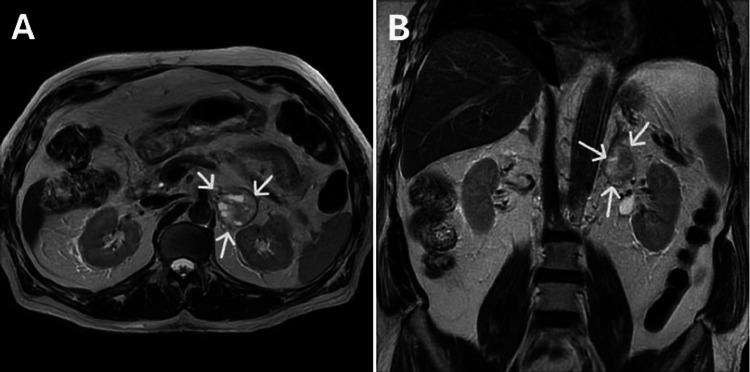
Magnetic resonance imaging scan of the abdomen. A: Magnetic resonance imaging scan of the adrenal glands (transverse view) demonstrating a lipid-poor left adrenal tumor, measuring 4.3 cm x 3.9 cm, and heterogenous in signal intensity (white arrows). B: Magnetic resonance imaging scan of the adrenal glands (coronal view) demonstrating the left adrenal tumor.
